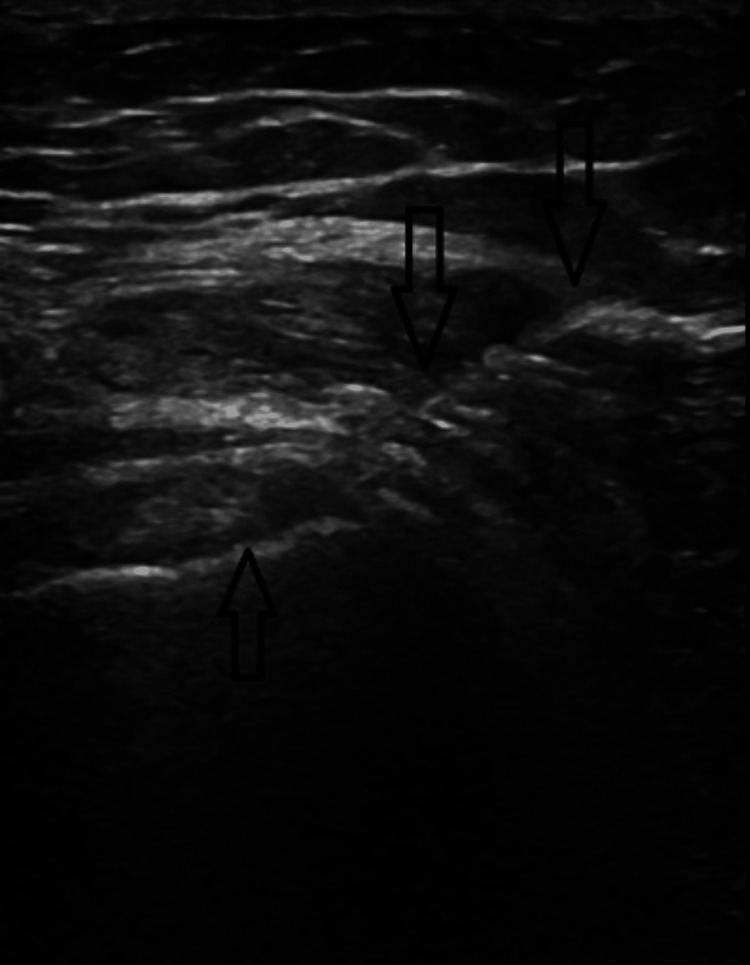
Snapshot of the ultrasound-guided methylprednisolone injection targeting calcific deposits on the supraspinatus tendon and the surrounding area, with arrows indicating the path of the needle.
